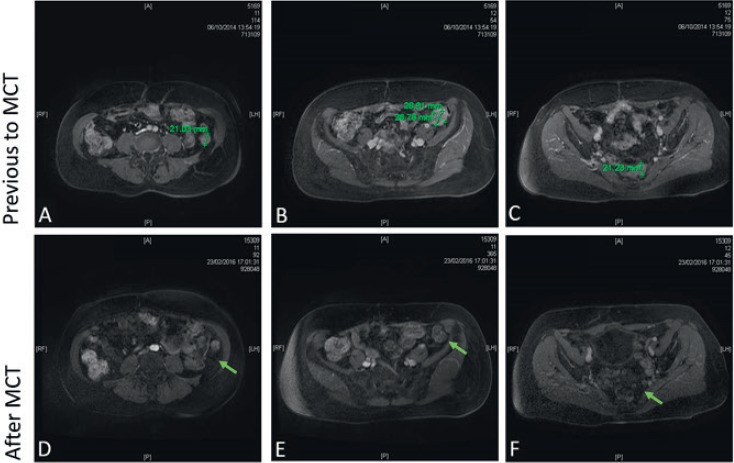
Contrast-enhanced pelvic magnetic resonance imaging. Previous MCT: (A) a 2.1 cm solid tumour in left paracolic grave, the tumour appears in close association with the rear edge of descendent colon and the left latero-conus fasia; (B) a 2.6 cm × 2.8 cm solid tumour lesion in contact with sigmoid colon; (C) a 2 cm tumour lesion in the pararectal space left rear. A, B, and C showed contrast enhancement after intravenous administration of gadolinium. After MCT: (D) no lesion in the left paracolic grave (arrow); (E) residual tumour lesion without diffuse heterogeneous enhancement with intravenous administration of gadolinium (arrow); (E) no lesion observed in the pararectal space (arrow).
